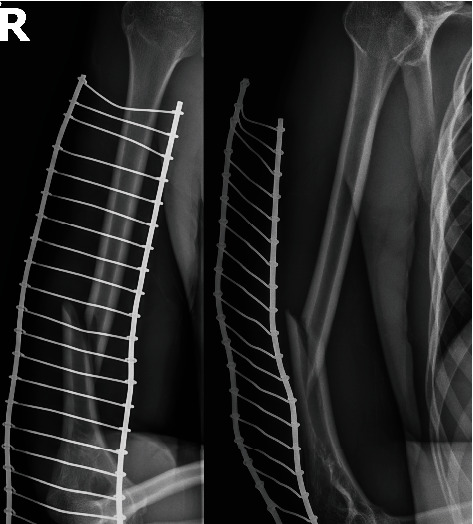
X-ray of the spiral humeral shaft fracture at distal third (12A1c-Holstein–Lewis fracture) usually not associated with severe radial nerve damage in children (figures from the Department of Trauma and Orthopedic Surgery database, Upper Silesian Children's Health Center, John Paul, Katowice, Poland).
